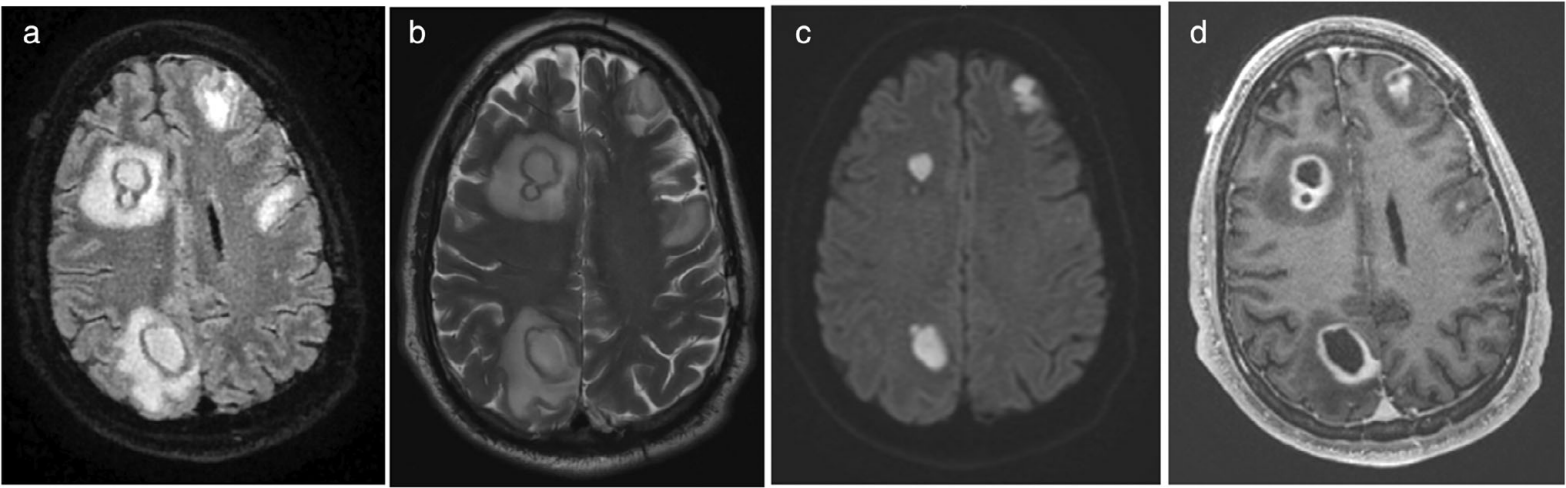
An example of bacterial infection: (a) Axial FLAIR image shows multiple cystic lesion characterized by a hypointense rim and surrounded by white matter hyperintensity. (b) Axial T2 weighted images shows signal characteristics similar to FLAIR. (c) Axial DWI based trace image shows restricted diffusion within the cystic lesions and (d) axial post gadolinium based T1 weighted image identifies thick rim surrounding cystic lesions which is significant for blood brain barrier disruption. (Courtesy John D. Port, Mayo Clinic, Rochester.)
